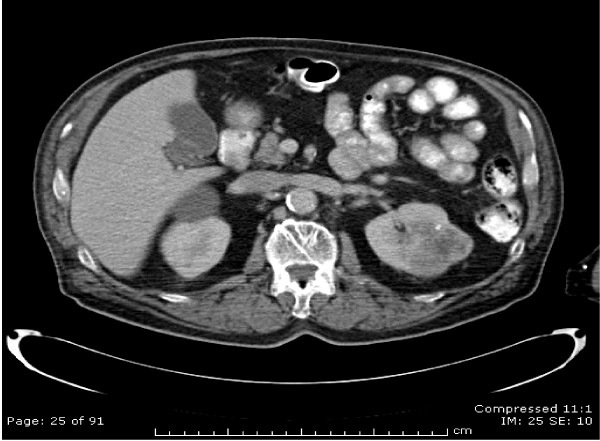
CT scan showing primary tumour of left kidney.
